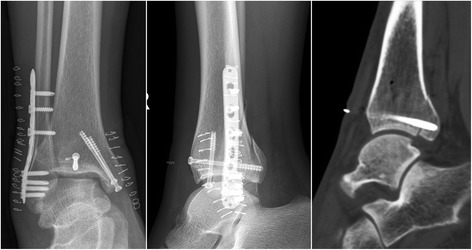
For the patient presented in Fig. 5, the PMF was reduced using ligamentotaxis following ORIF of lateral and medial malleoli. Percutaneous screw fixation of PMF was achieved through a stab incision. The postoperative sagittal reconstruction of CT images provided more precise determination of residual articular step-off of the posterior malleolus which could be easily ignored in radiographs
